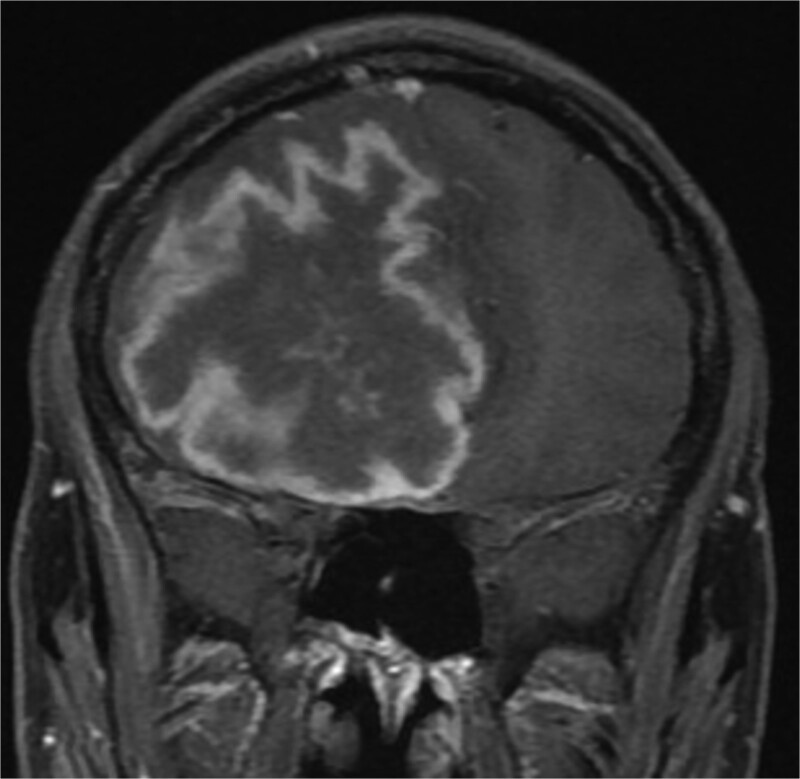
Brain MRI showing right-sided cerebral abscess with midline shift to the left side.
